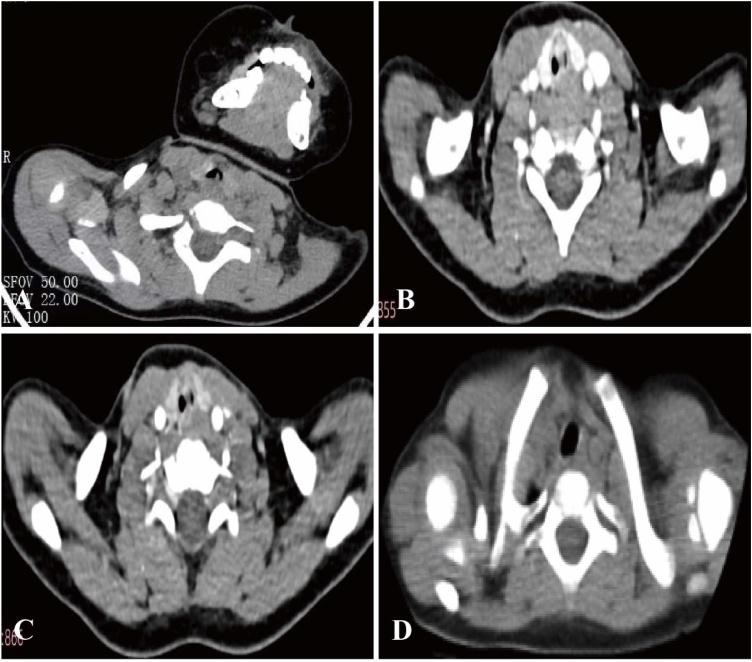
Chest computed tomography and computed tomography angiography images. (A) A nodular high-density lesion is noted at the subglottic-tracheal junction. (B) Venous phase: A nodular high-density lesion along the medial wall of the left trachea (at thyroid gland level) demonstrates mild density inhomogeneity. (C) Arterial phase: The lesion shows marked enhancement. (D) No evident mass lesion is identified within the trachea.
